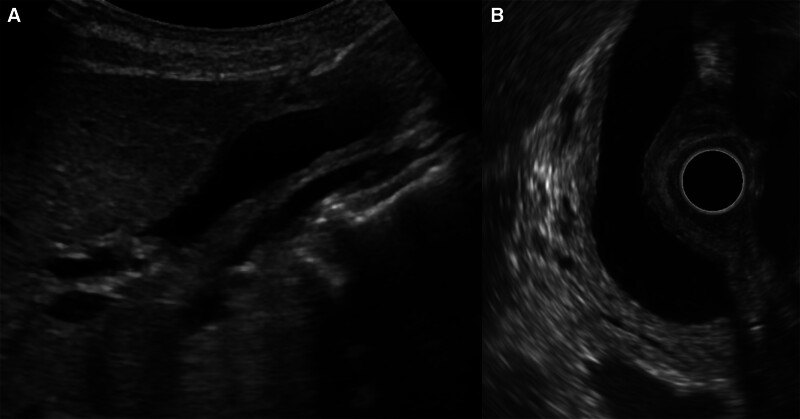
Abdominal ultrasonography revealing wall thickness of the right hepatic duct and upper bile duct (A). Endoscopic ultrasonography showing wall thickening up to the lower bile duct without evident tumor lesions (B).
